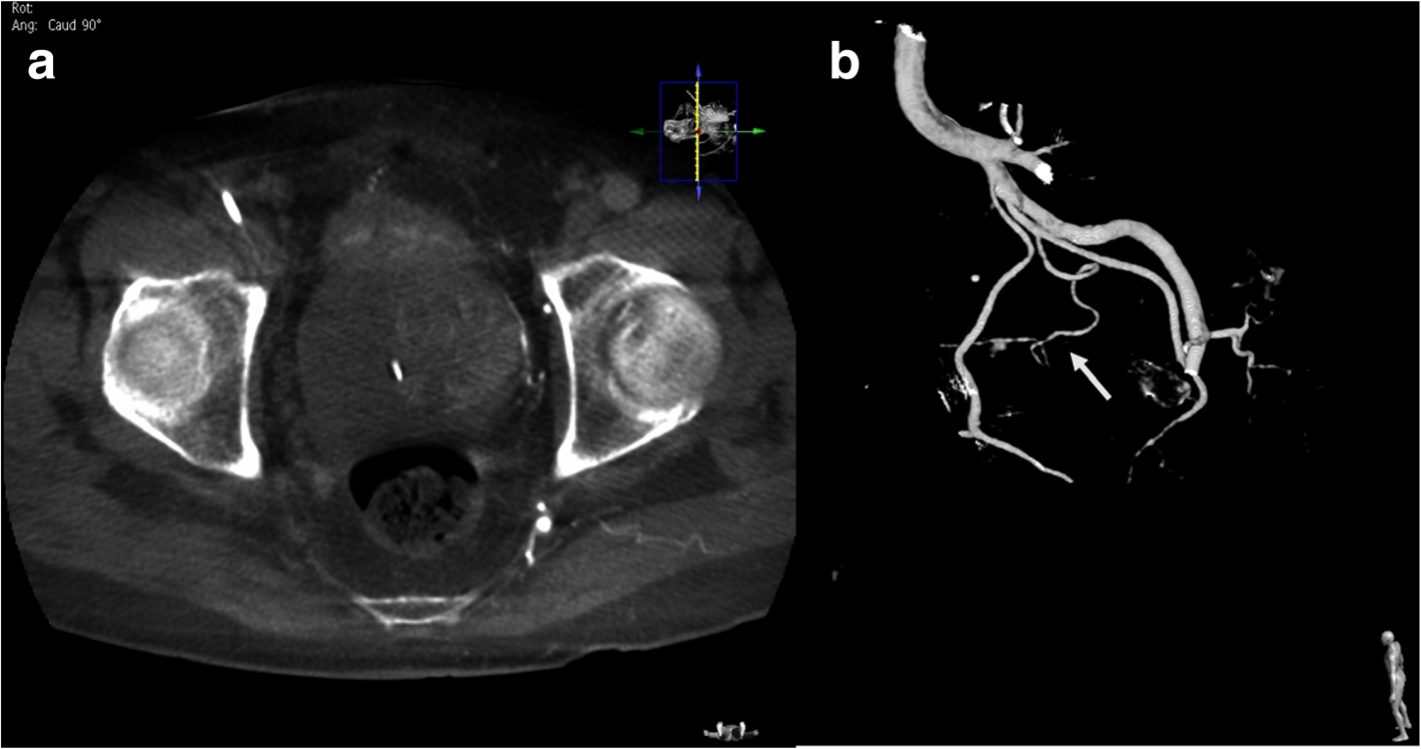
Contrast CBCT of the left internal iliac artery. a Good parenchymal enhancement of the left aspect of the prostate gland was visualized. b Three-dimensional reconstructed image demonstrates the vascular anatomy of the LPA (arrow)
